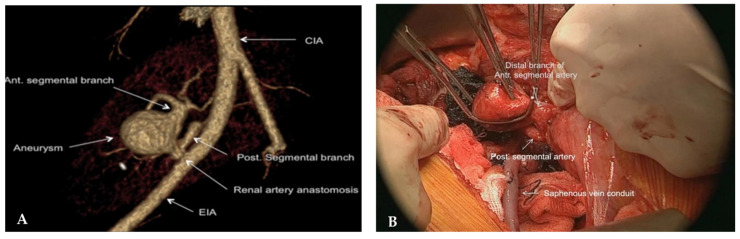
CT angiogram 3D reconstruction (A) and intraoperative image (B) demonstrating renal artery aneurysm. (A) Arterial aneurysm of the anterior segmental branch seen on 3D CT angiogram. (B) Intraoperative image of renal artery aneurysm involving the anterior segmental branch. CIA: common iliac artery, EIA: external iliac artery. Image reused from “Successful repair of transplant renal artery aneurysm (TRAA)”, by Ho CERH et al., published by BMC Urology, accessed on 18 December 2025, licensed under CC BY 4.0 and CC0 1.0 Universal (http://creativecommons.org/licenses/by/4.0/ and https://creativecommons.org/publicdomain/zero/1.0/) [32]. These figures were cropped and merged from the original article to create this new single figure. Labels (A,B) were not in the original figure. Minor modifications were made to the title and descriptive captions.
